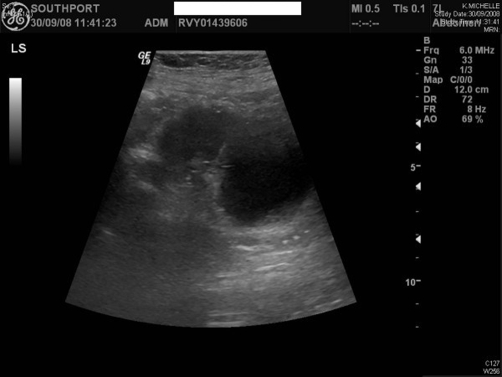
Sagittal ultrasound scan of urinary bladder, performed on 30 December 2008: The two compartments of hourglass bladder are seen.
